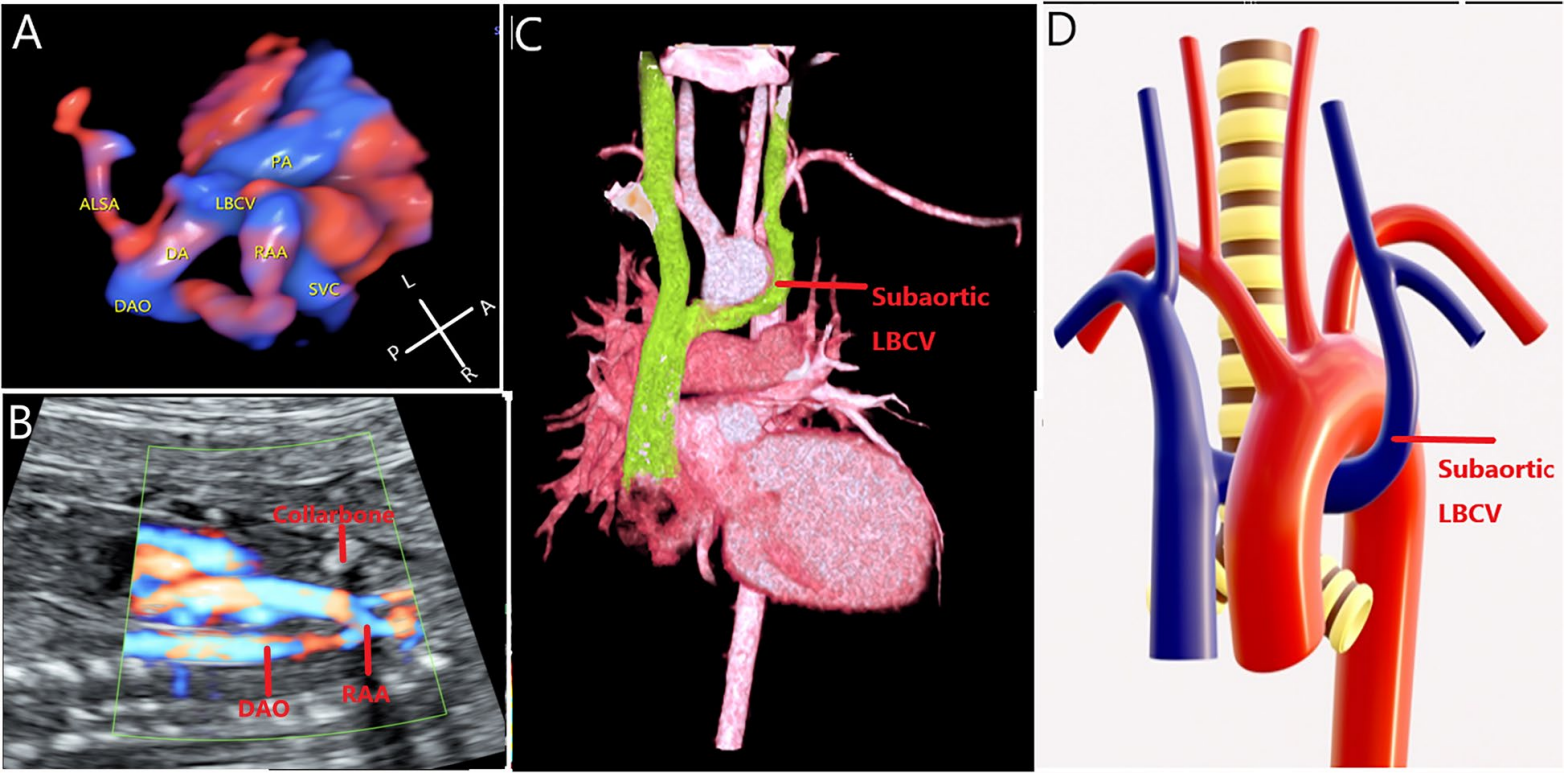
Prenatal and postpartum images of the subaortic LBCV. A Three-dimensional high-definition flow render mode and STIC are used to show the subaortic LBCV in a fetus. B Cervical aortic arch corresponds to the level of the collarbone. C Postpartum CTA showing that the subaortic LBCV, which was consistent with the prenatal image. D Structural presentation of subaortic LBCV. LBCV, left brachiocephalic vein; RAA, right aortic arch; PA, pulmonary artery; SVC, superior vena cava; DA, ductus arteriosus; DAO, descending aorta; LBCV, left brachiocephalic vein; L, left; R, right; A, abdominal; P, spinal
